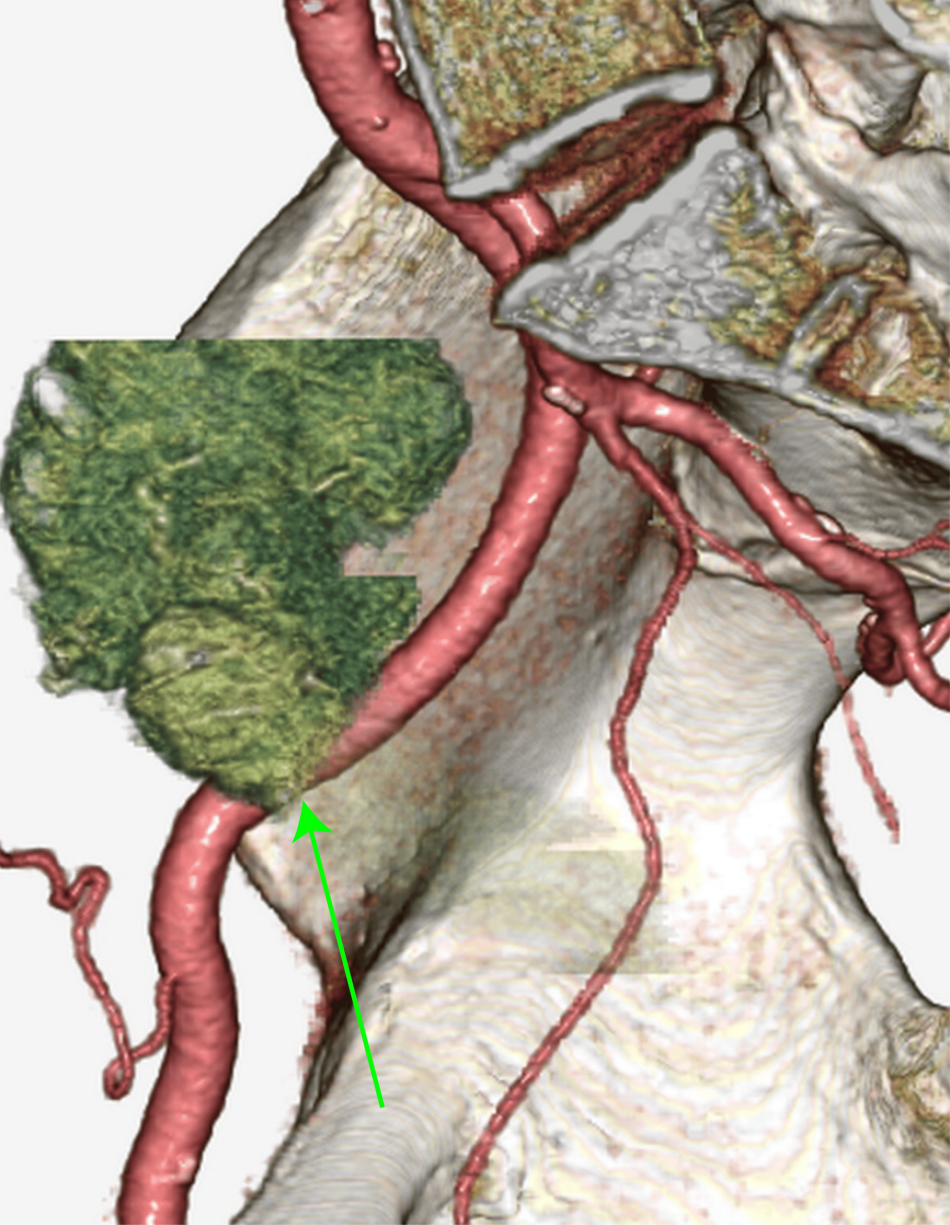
CT image (volume rendered) after chemotherapy shows that the extent of tumor infiltration into the right external iliac artery has decreased (green arrow).
